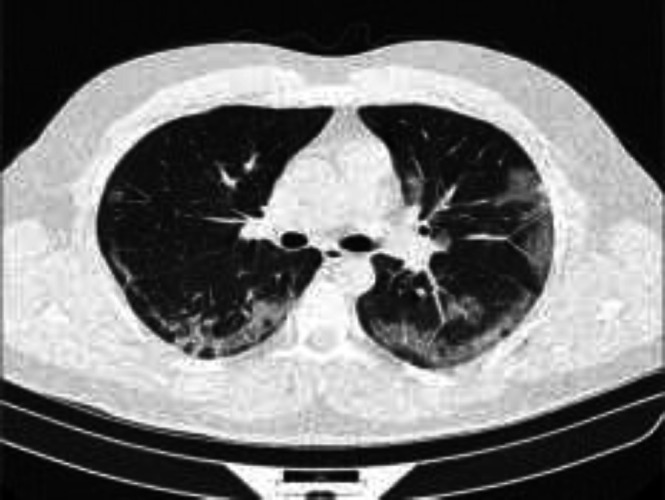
A 62-year-old female patients with chief complaint of dyspnea, fever, and cough showing bilateral ground-glass opacities in chest CT
